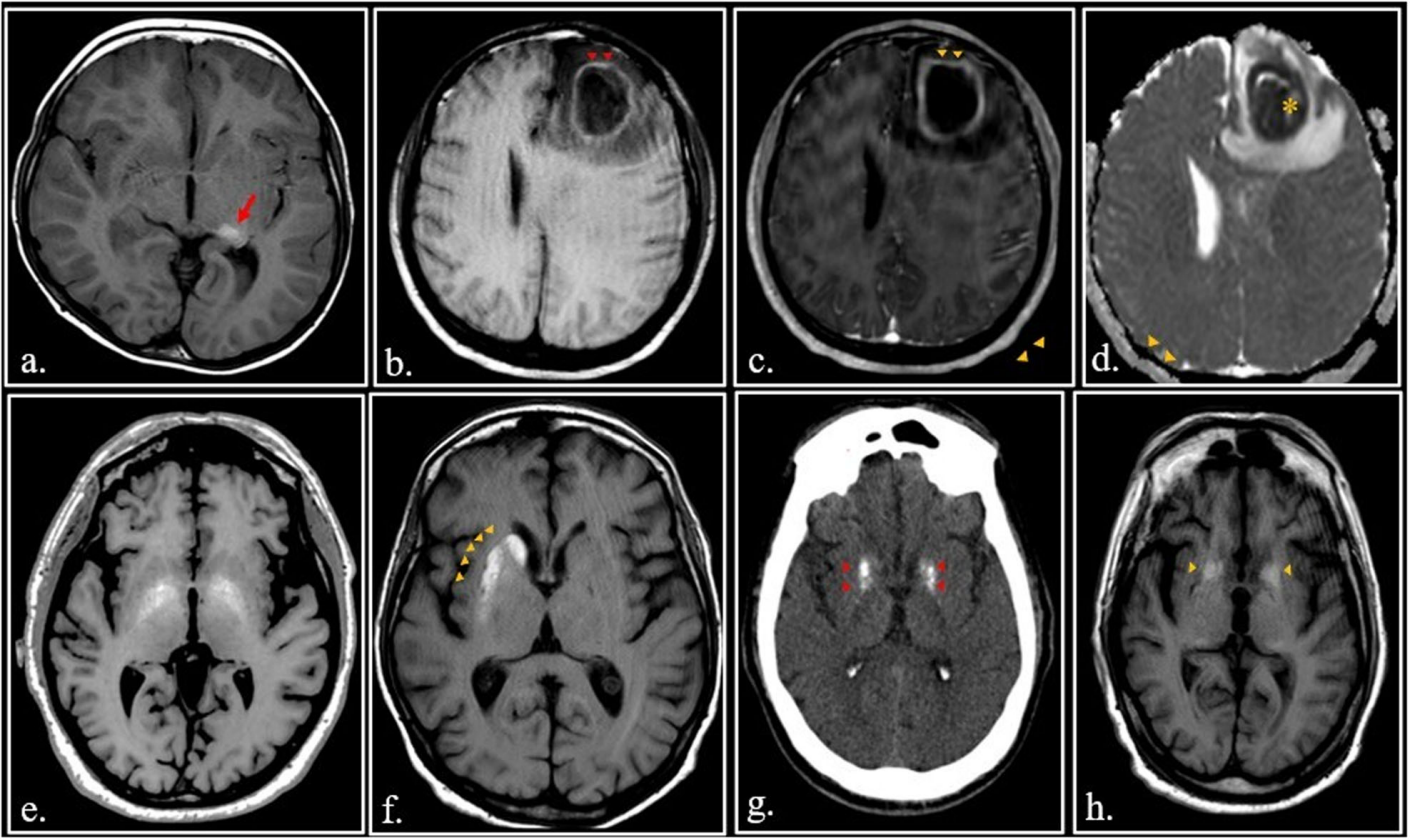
a 7-year-old girl with leptomeningeal and intraparenchymal melanosis. Axial SE T1-weighted image shows an hyperintense melanin-containing nodule in the left posterior hippocampus (arrow) (b–d) A high signal ring (red arrowheads) can be seen on unenhanced FSE T1- weighted scan in a left frontal brain abscess (a) with intense enhancement (yellow arrowheads) after Gadolinium administration (b). Apparent Diffusion Coefficient (ADC) map (c) shows the typical restriction (asterisk) of the abscess. e 62-year-old man with hepatic failure and parkinsonism. FSE T1-weighted image shows bilateral hyperintensity of globus pallidus (arrowheads). f 58-year-old diabetic female with a rapid onset of hemichorea-hemiballismus. Marked hyperintensity of the right putamen and caudate nuclei (arrowheads) is visible on unenhanced FSE T1-weigthed image. g, h 64 years-old psychiatric patient with Fahr disease. CT scan (a) shows bilateral calcification of the globus pallidus (red arrowheads). On FSE T1-weighted image (b), the calcification appears hyperintense (orange arrowheads)
